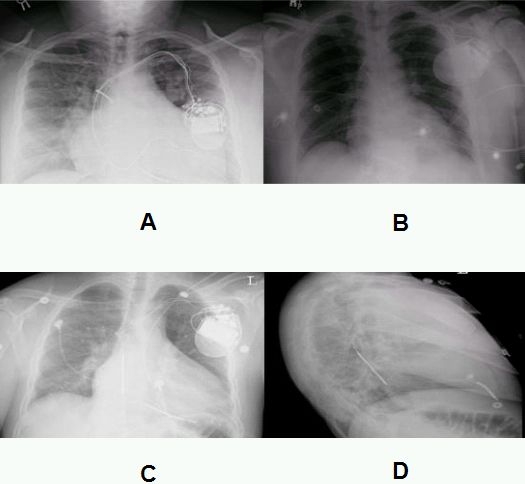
Chest X-ray showing the position of azygous vein lead
